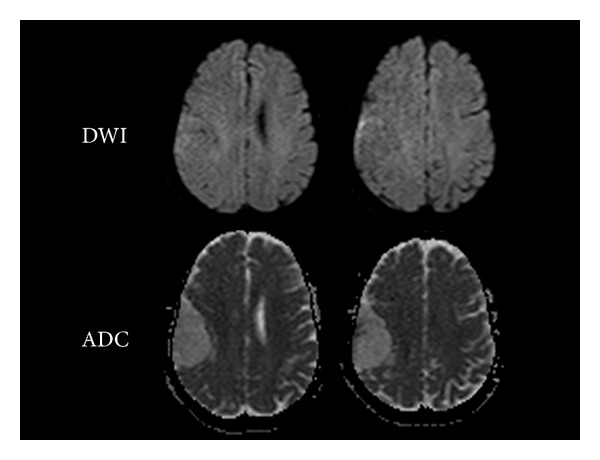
Diffusion-weighted image (DWI) and apparent diffusion coefficient (ADC) map image. The cystic portion of the lesion shows facilitated diffusion, although slightly more restricted than CSF.
